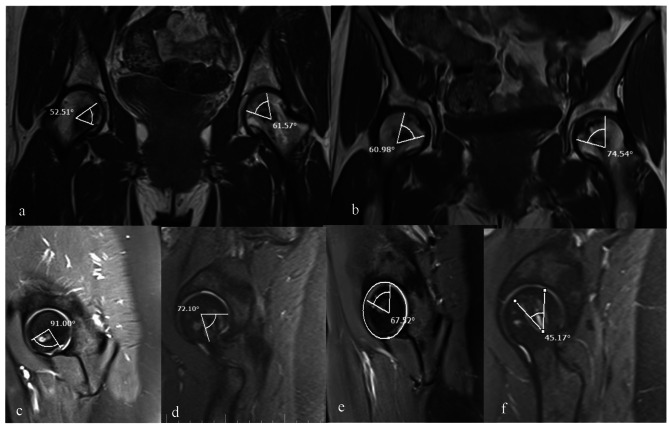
Preoperative (a) and (b) postoperative MRI, preoperative (c) and (d) postoperative MRI of right femoral head, preoperative (e) and (f) postoperative MRI of left femoral head
